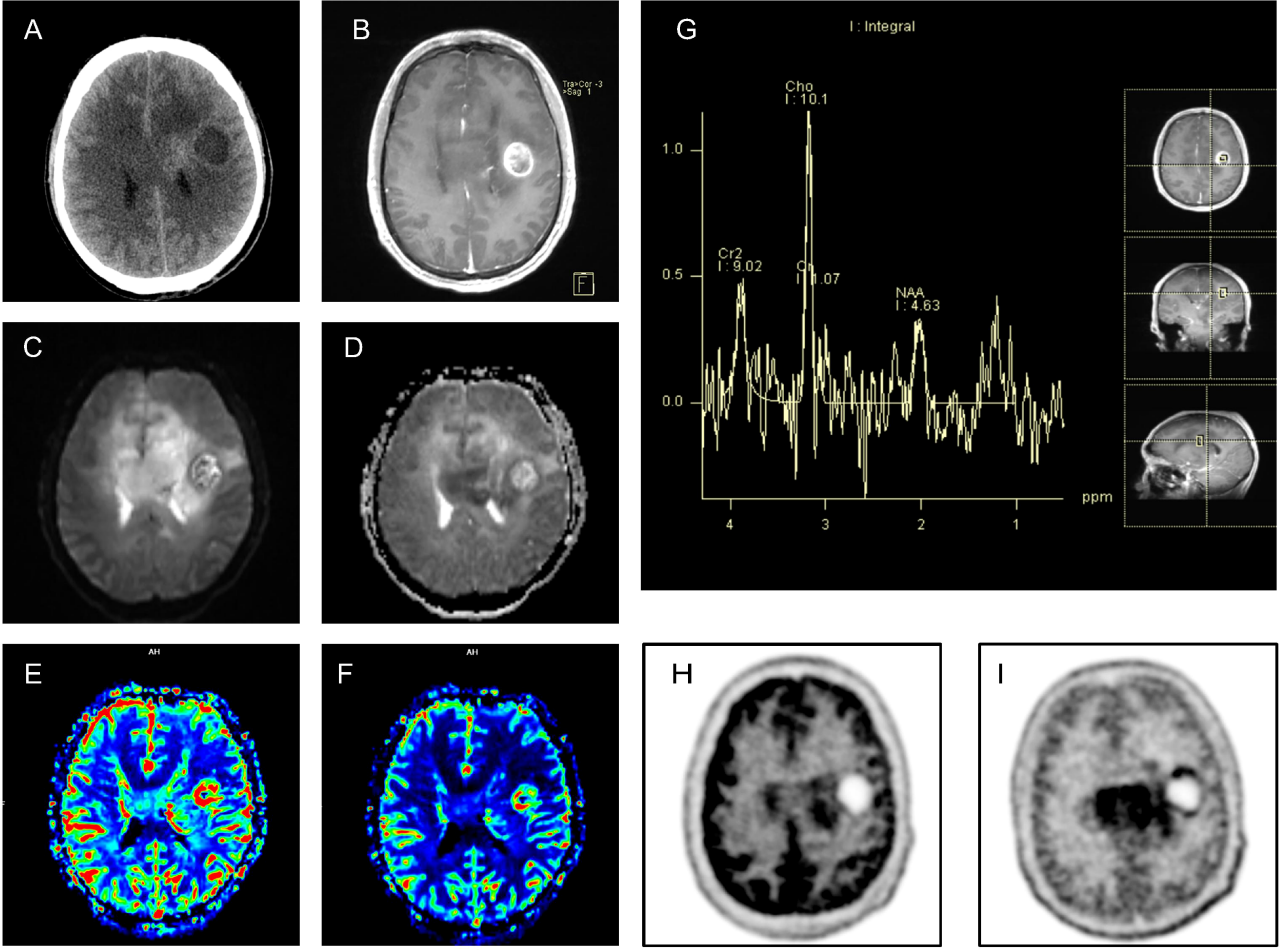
The patient’s cranial imaging demonstrates features consistent with malignant tumor lesions: Panel (A), cranial CT, reveals a pronounced mass effect, midline shift, lateral ventricle compression, and a focal area within the left corpus callosum demonstrating increased density; Panel (B), contrast-enhanced MRI sequence, shows a nodular lesion with complete ring-like enhancement and heterogeneous internal enhancement within the lesion; Panels (C, D), DWI and ADC sequences, demonstrate marked diffusion restriction in the corpus callosum and left periventricular region (hyperintensity on DWI, hypointensity on ADC); Panels (E, F), PWI sequence CBV and CBF maps, reveal increased perfusion in the nodular lesion and left periventricular region; Panel (G), MRS analysis, shows an elevated Cho/NAA ratio in the lesion site, reaching 2.18; Panels (H, I), PET-MRI with radioactive tracers ^18F-FDG and ^11C-MET, indicate significantly increased uptake of both tracers.
